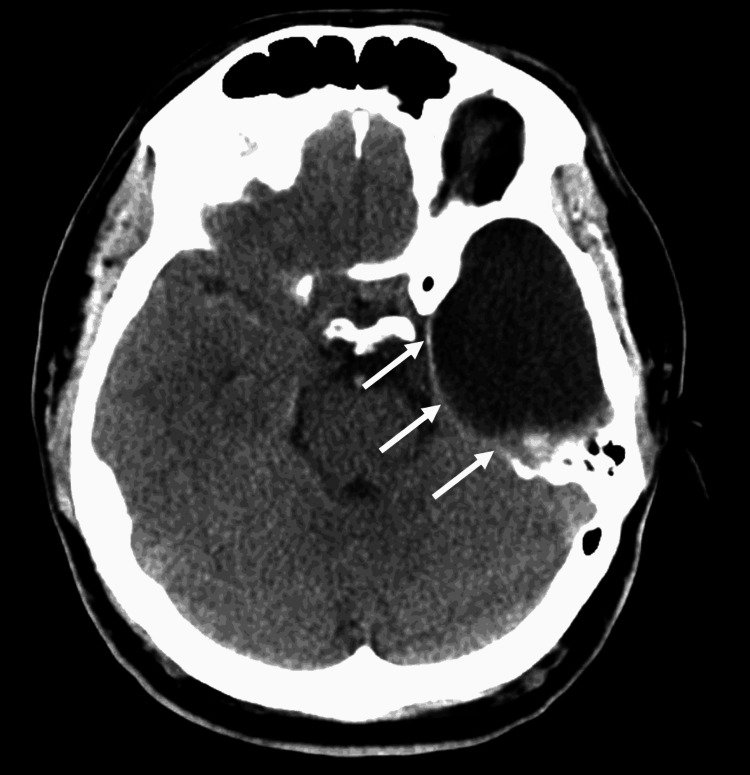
MRI of the head revealed a large left-sided arachnoid cyst (arrows) and redemonstrated the right-sided MCA stroke. MCA: middle cerebral artery; MRI: magnetic resonance imaging
